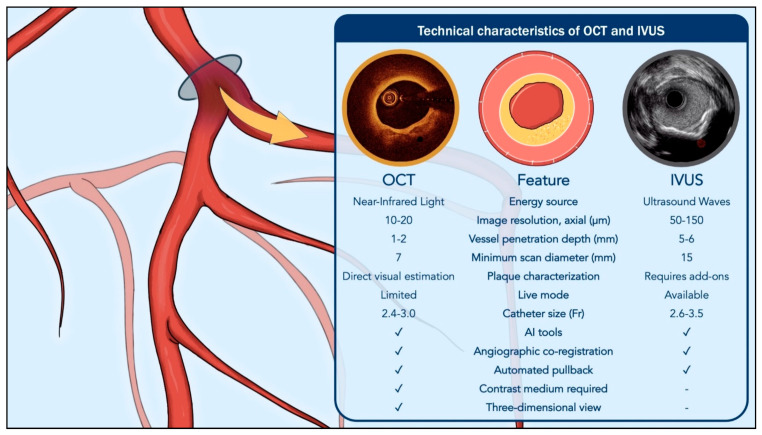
Comparative technical characteristics of optical coherence tomography and intravascular ultrasound. Abbreviations: AI, Artificial Intelligence; IVUS, Intravascular Ultrasound; OCT, Optical Coherence Tomography.
